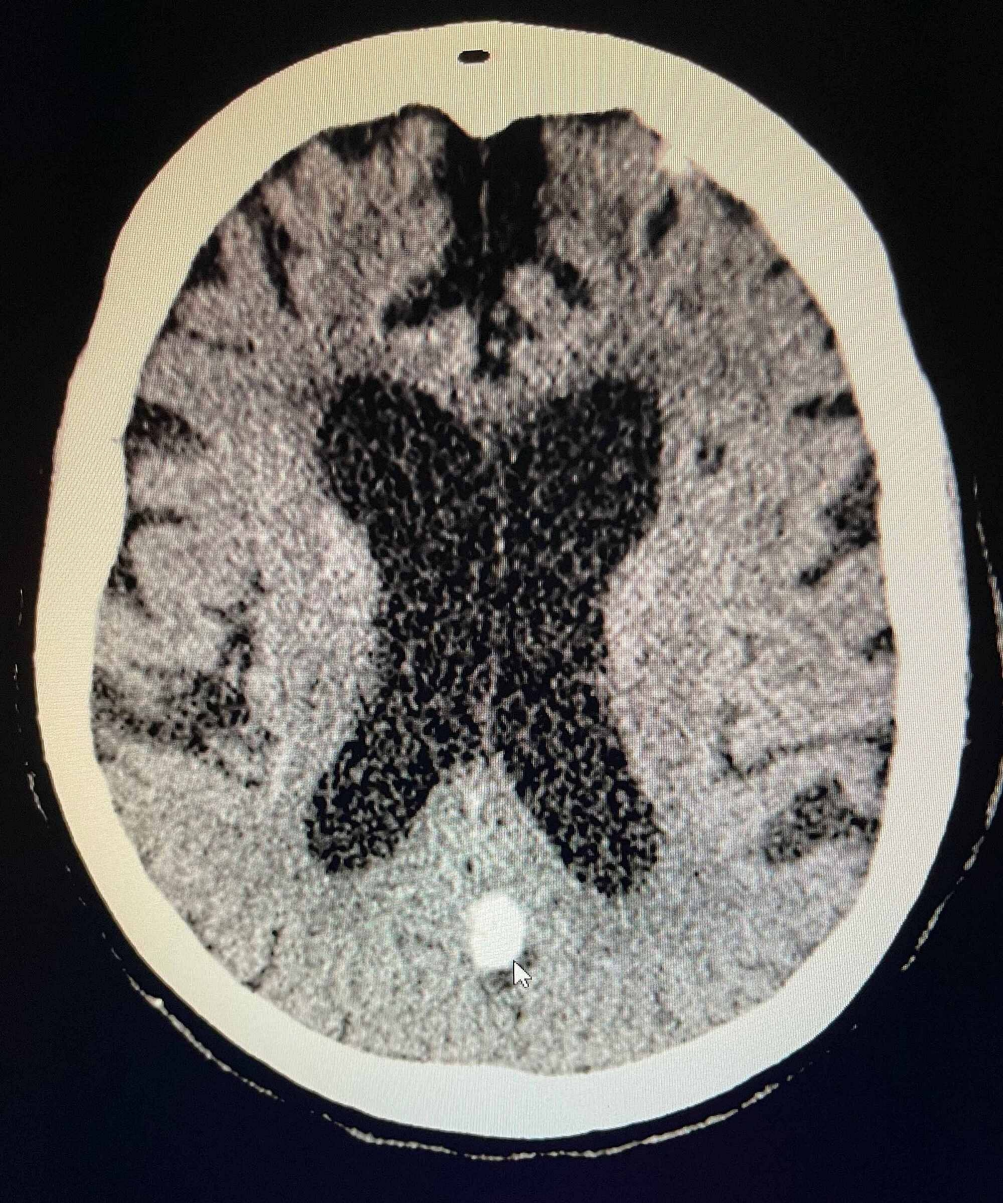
Computerized tomography (CT) scan of the head showing volume loss with disproportionate involvement of medial temporal lobes and a 12 mm calcified probable meningioma of left parafalcine region.
